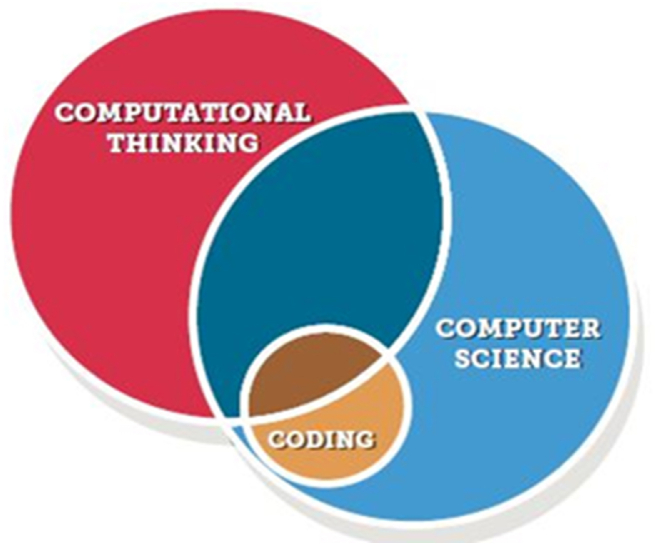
The relationship between coding, computer science and CT [24].
